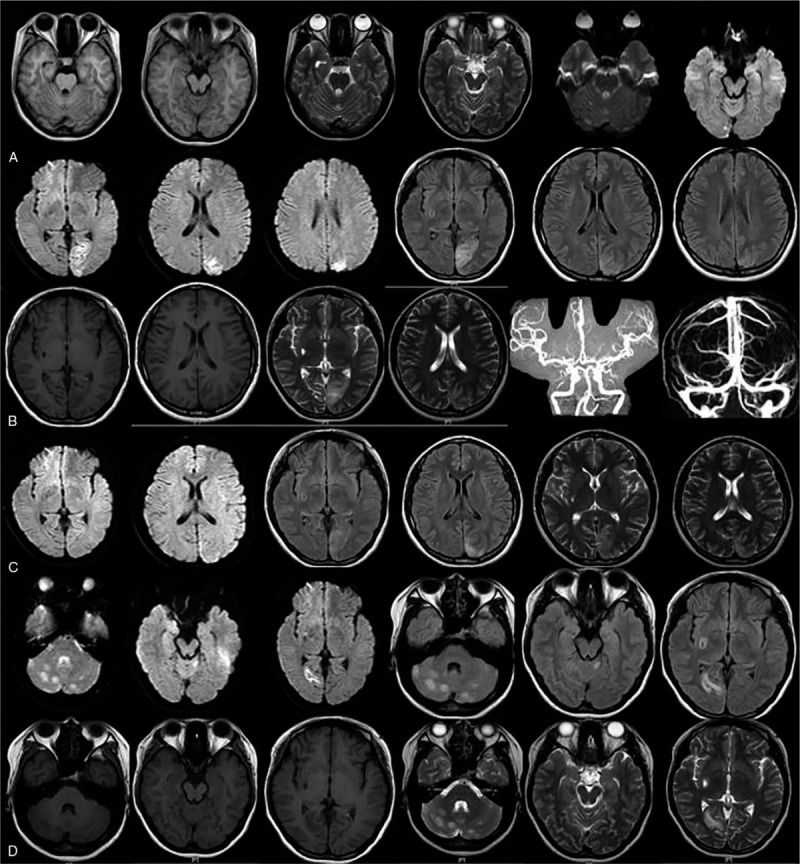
Image data of brain. A: The first hospitalization at 19 years old. Brain MRI showed punctiform long T2 and T1 signals at right cerebellar hemisphere and right occipital lobe, unclear boundary and DWI images with hyperintensity. B: At 21 years old. Brain MRI showed gyrus-shape long T1 and T2 abnormal signals at left-side pillow top and FLAIR and DWI images with hyperintensity. Brain MRV and CTA in head and neck showed no obvious abnormity. C: Reexamination after 10-day treatment. Brain MRI showed that gyrus shape long T1 and T2 signals at left occipital parietal lobe was improved. D: Recurrence at 22 years old. Brain MRI showed schistose and patchy signals at cerebellar hemispheres, vermis cerebelli, and right occipital area. MRI = magnetic resonance imaging.
